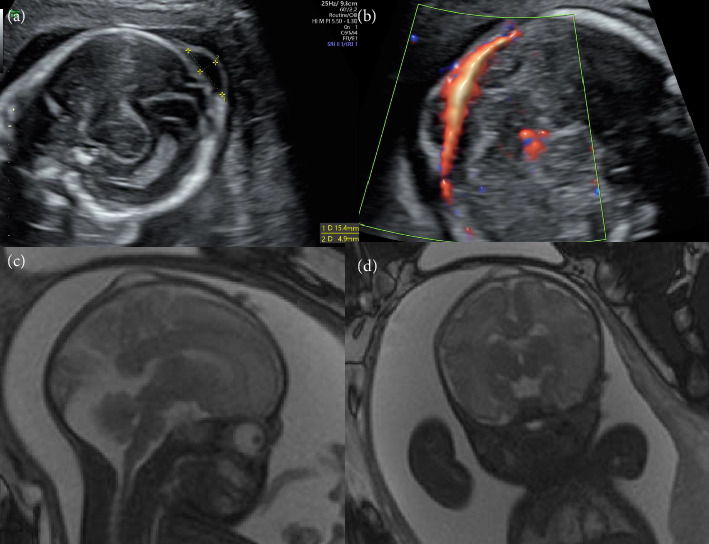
The cyst observed under the scalp of the fetus using two-dimensional ultrasonography and magnetic resonance imaging (MRI). (a) Image obtained using two-dimensional ultrasonography at 20 weeks of gestation. A 15 × 5 mm fetal scalp cyst and a vague border between the cyst and fetal skull bone can be seen. (b) Image obtained using two-dimensional ultrasonography with power Doppler at 22 weeks of gestation. No blood flow from the intracranial space to the scalp cyst could be observed; however, blood vessels were present. (c) Image of the lateral view obtained using T2-weighed MRI at 32 weeks of gestation. A border between the scalp cyst and fetal skull bone can be seen. (d) Image of the coronal view obtained using T2-weighed MRI at 32 weeks of gestation. A border between the scalp cyst and fetal skull bone can be seen.
